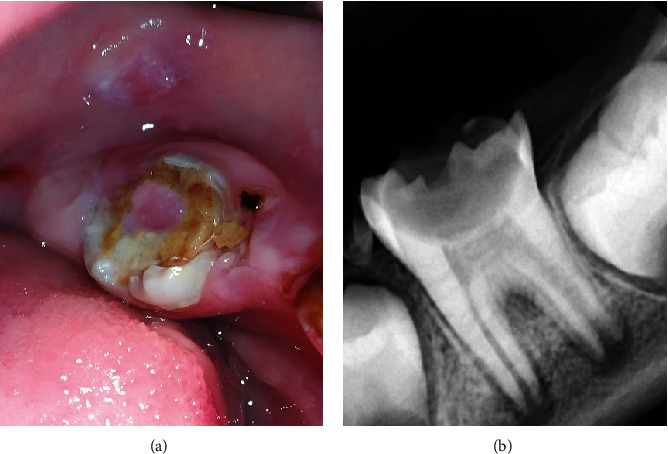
The lower left first permanent molar: (a) initial intraoral image and (b) preoperative periapical radiograph.
